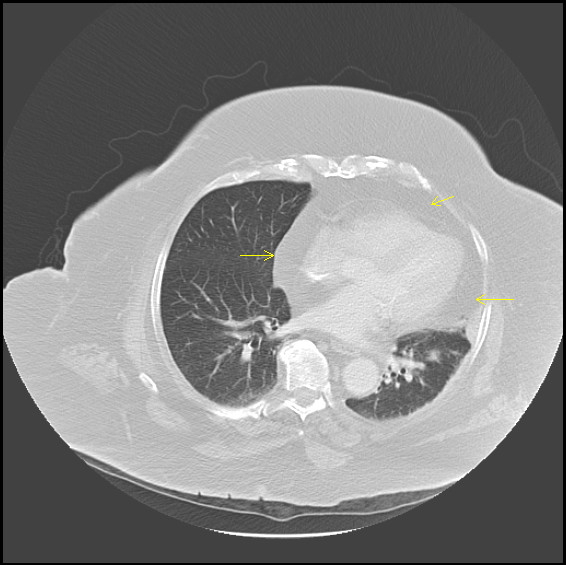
Computed tomography scan of the chest showed presence of significant fat in the mediastinum surrounding the heart and other mediastinal structures.
